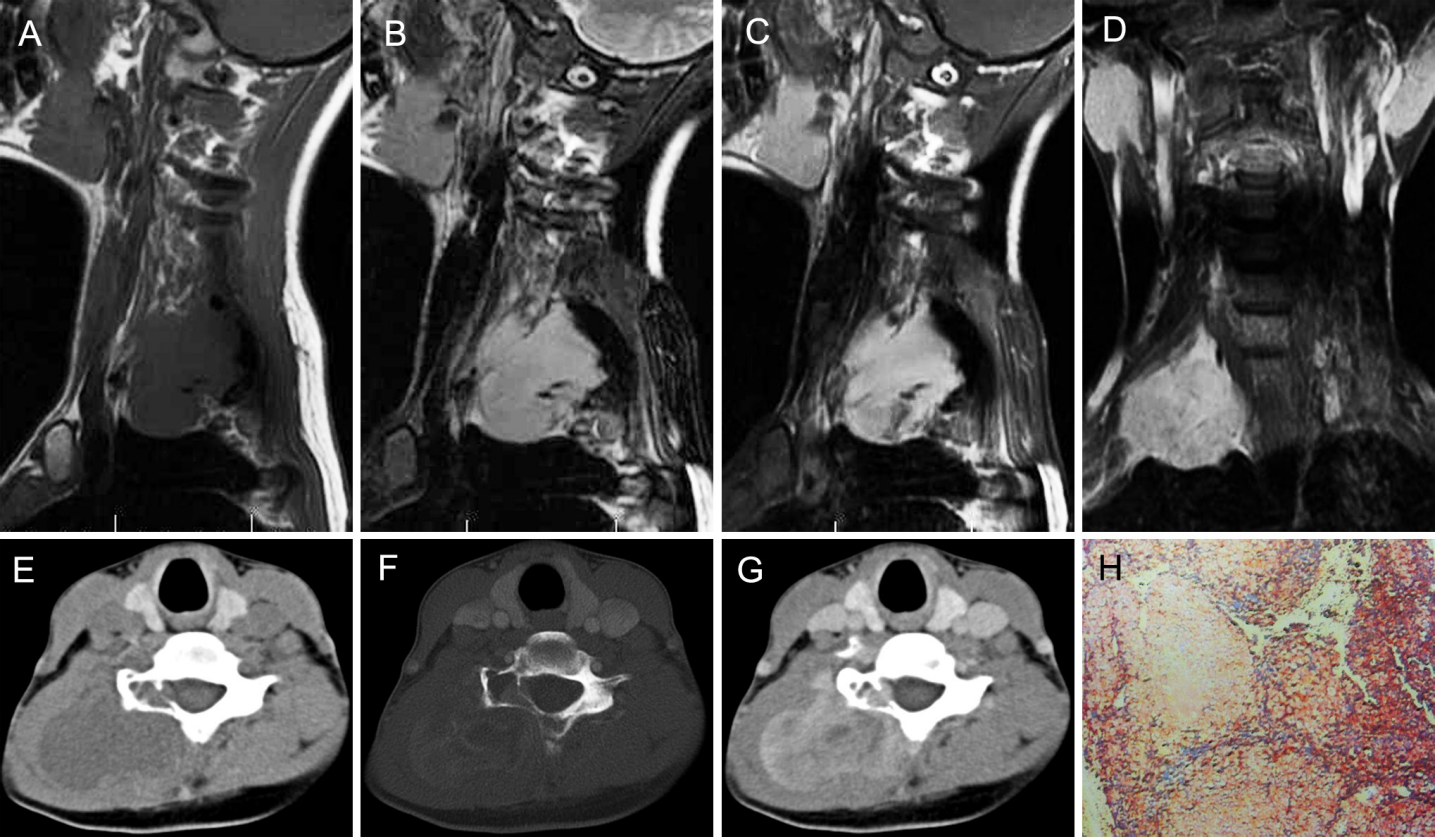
pPNET in a 20-year-old woman Precontrast MRI showed a lobulated soft tissue mass in the right neck that was iso-intense on T1WI (A) and hyper-intense on T2WI (B). Contrast MRI showed the mass had significant and heterogeneous enhancement (C). Enhanced coronal MRI (D) showed a mass with poorly defined margins. Precontrast CT images showed a large low-density mass with a well demarcated margin (E). Bone windows showed destruction of the right vertebrae (F). Contrast CT images showed the mass had slight heterogeneous enhancement (G). The poorly differentiated small round tumor cells were CD99 positive (H × 100).
